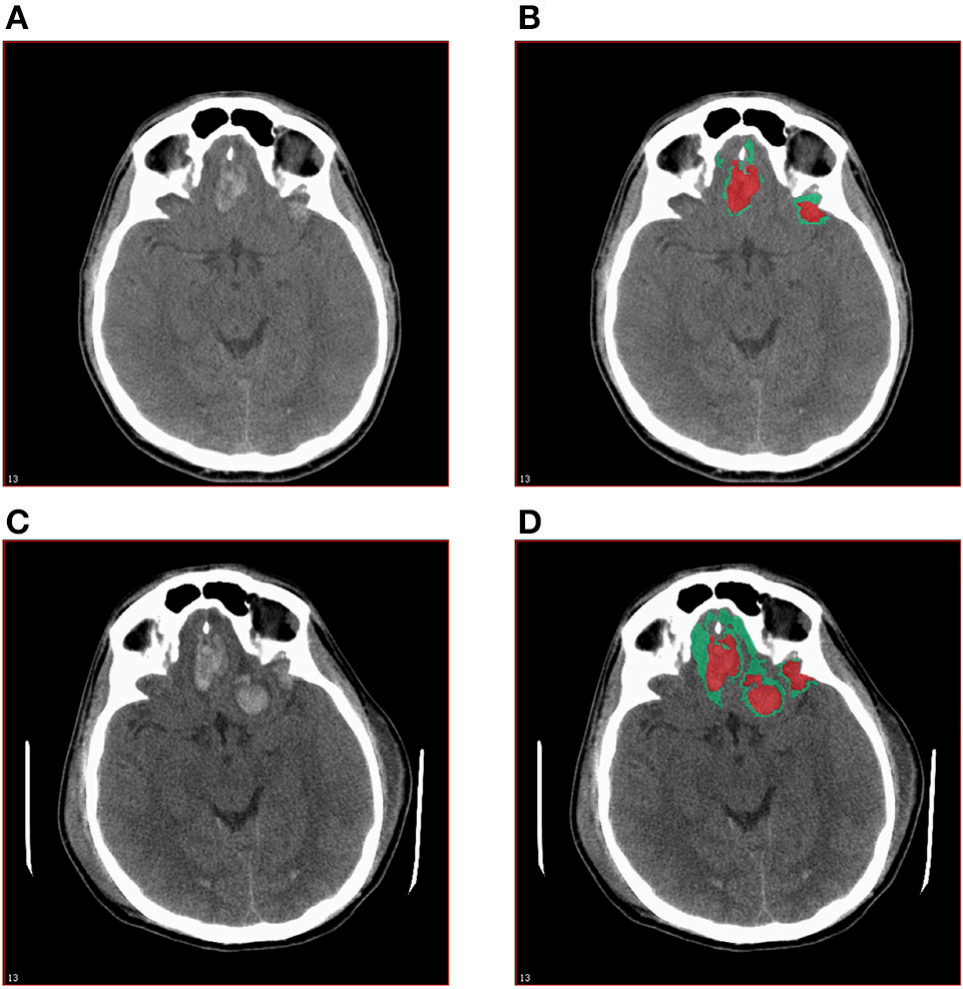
Quantitative measurement of high density and low density components of traumatic ICH. The base layer (A) and annotated (B) images of the initial CT scan and the base layer (C) and annotated (D) images of the CT scan at 12–36 h post-injury are shown.
